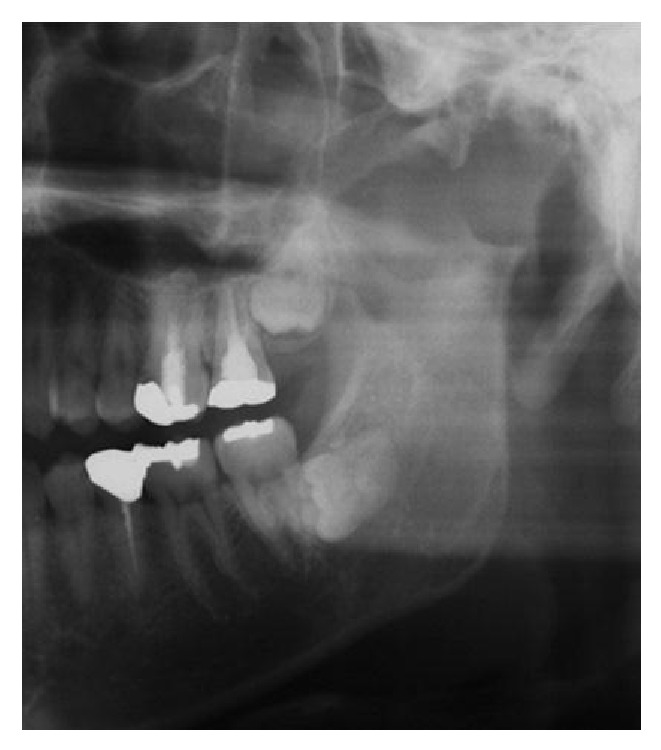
Bone resorption from left fossa to condyle in panorama radiograph.
